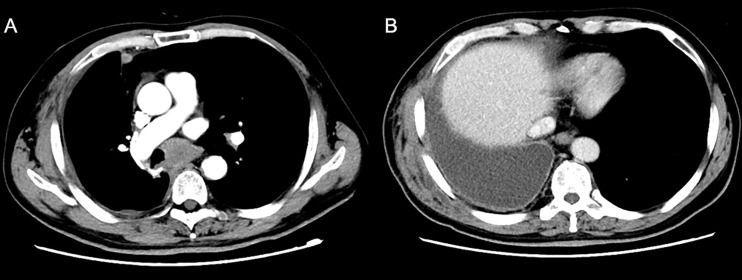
A reexamination CT scan one month after surgery showed new soft tissue nodules in the mediastinum.
